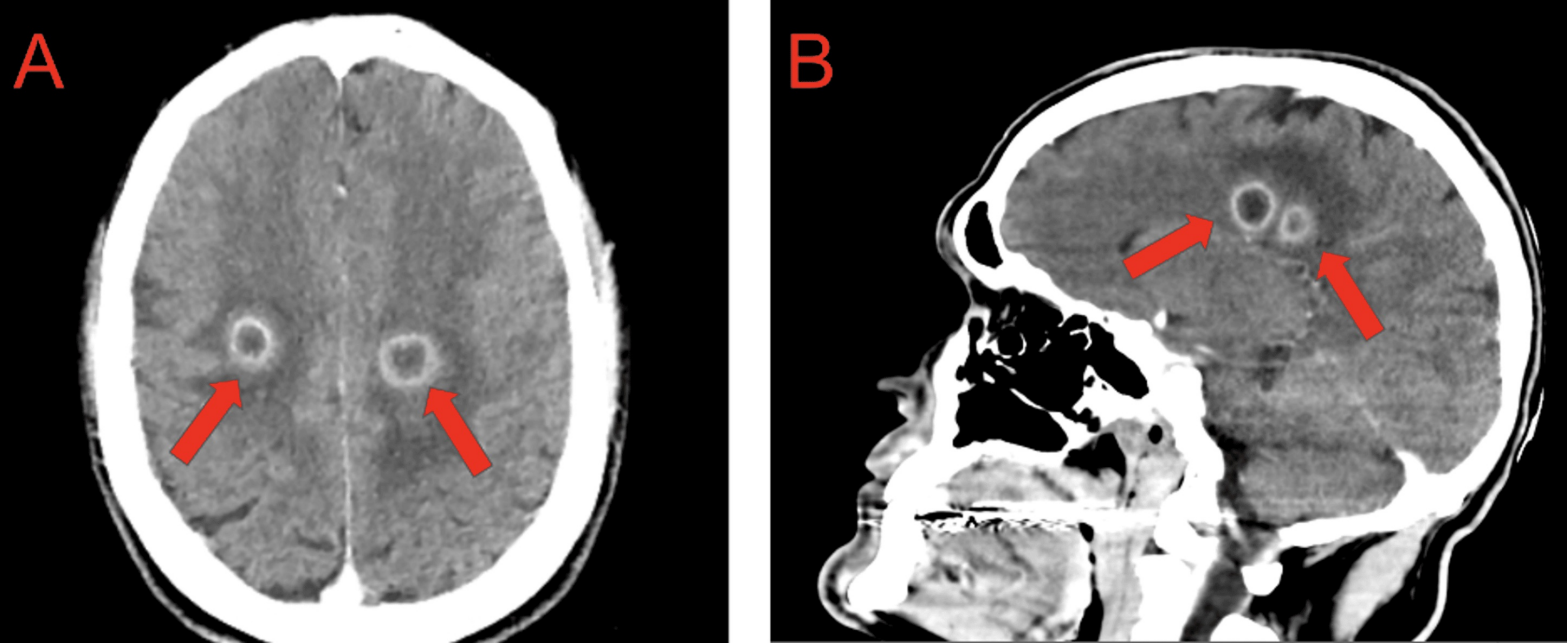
(A) Axial and (B) sagittal CT scans of the head with contrast taken in the emergency department, clearly showing ring-enhancing lesions consistent with abscesses.
